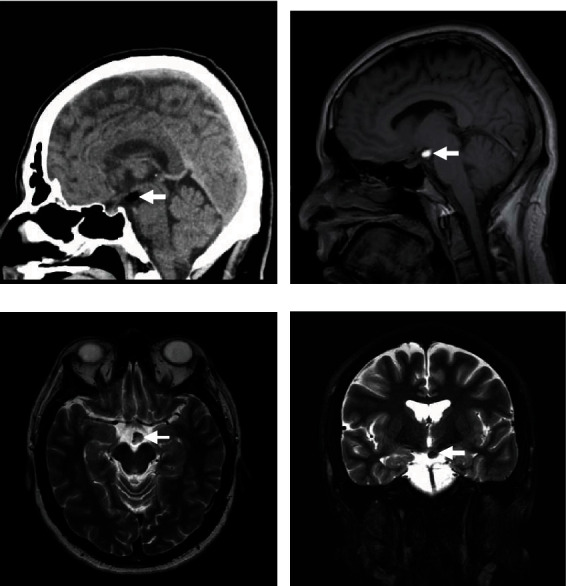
(a) Sagittal brain CT without contrast. (b) Sagittal T1-weighted MRI. (c) Axial T2-weighted MRI. (d) Coronal T2-weighted fat-saturated MRI. (a)–(d) The images demonstrate a homogeneously oval-shaped, fat-containing suprasellar hypothalamic lesion measuring 11 × 7 × 7 mm in the anterior-posterior, transverse, and craniocaudal dimensions, respectively, on the left side. The lesion is hyperintense in T1-weighted images, intermediate to hyperintense in T2-weighted images, and suppressed on fat-saturated images. No internal septation, nodularity, or calcifications are noted.
